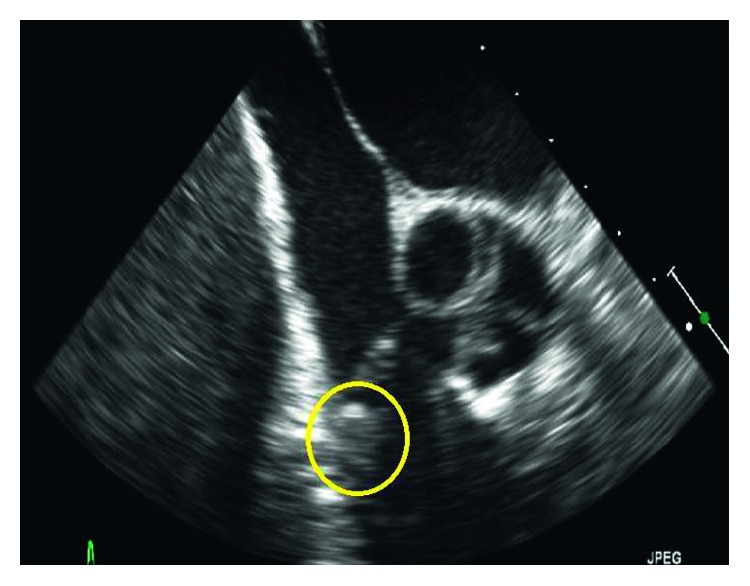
Transesophageal echocardiogram displaying a 10 mm mobile mass appreciated on 2D and 3D views consistent with vegetation (yellow circle).
